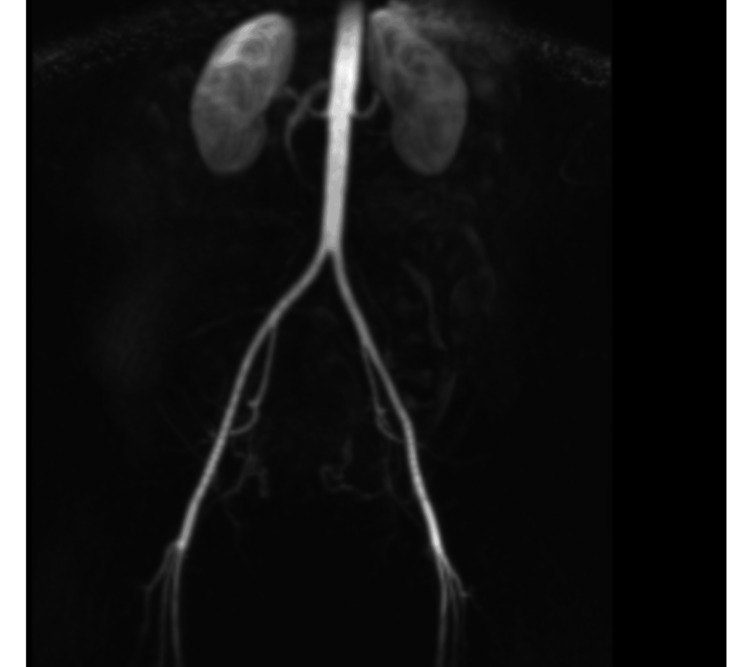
MRI image of dynamic contrast enhanced pelvic MRI angiogram depicting opacification of bilateral uterine arteries with no obvious variant uterine arterial supply
